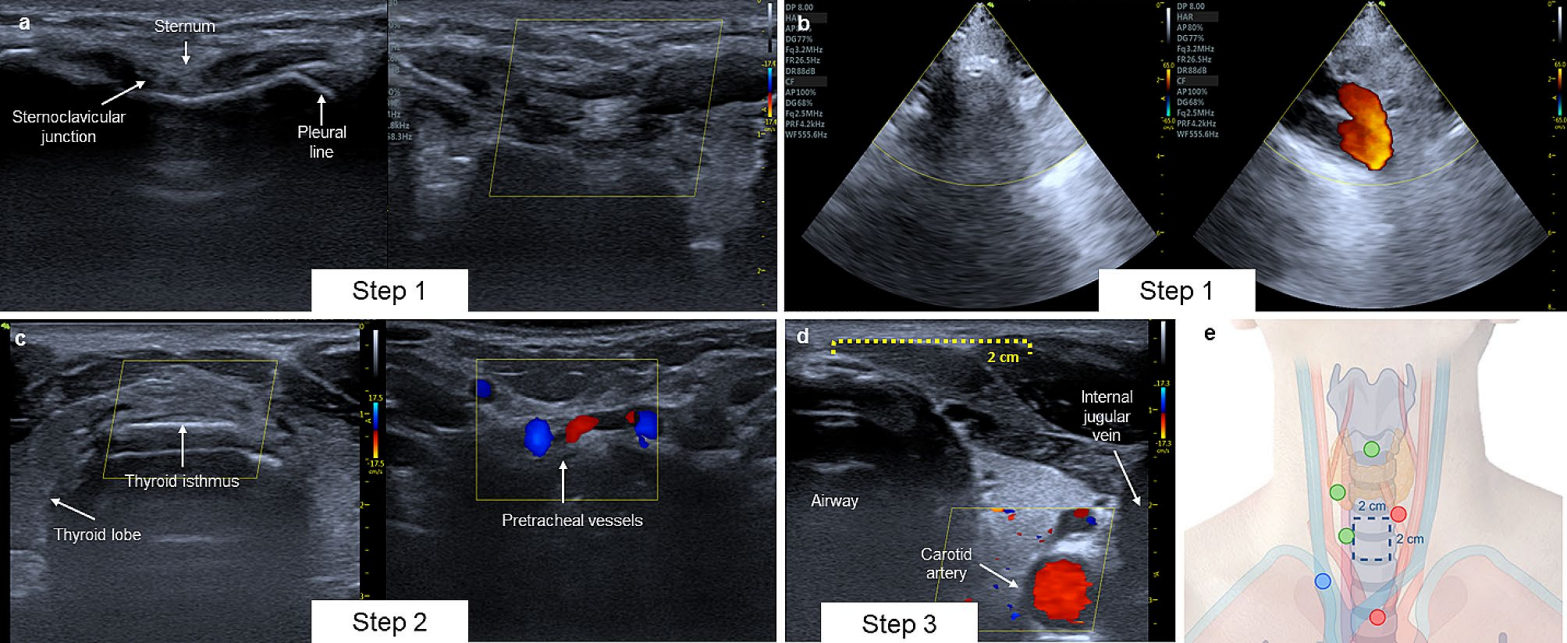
A Step 1: ultrasound exploration with linear transducer, absence of high-riding innominate artery. B Step 1 ultrasound exploration with sector transducer, innominate artery is identified with color Doppler. C Step 2: pretracheal structures identified with color Doppler along the transversal axis of the airway. D Step 3: Lateral margins are defined measuring the distance from the midpoint of the trachea to the lateral margin of the vessel. E The safety area is delimited (Created with BioRender.com)
